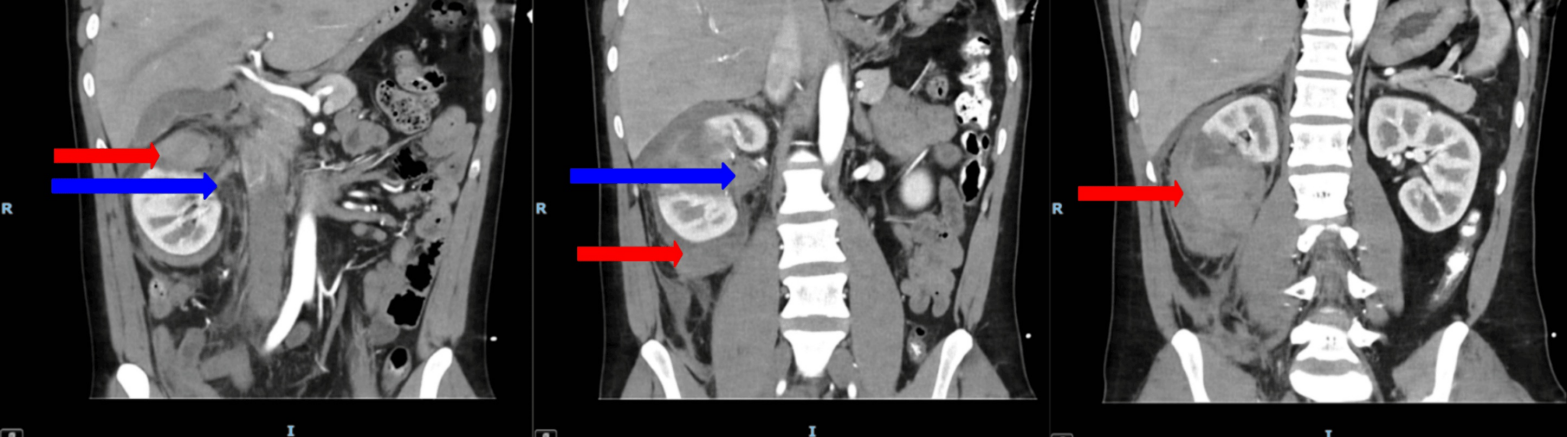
CT angiogram renal (coronal view, series) on day two of hospital admission The red arrows point to the perinephric hematoma. The blue arrows point to renal hilum disruption. The letter R depicts the right-hand-side of the patient. The letter I depicts the inferior aspect of the patient.
